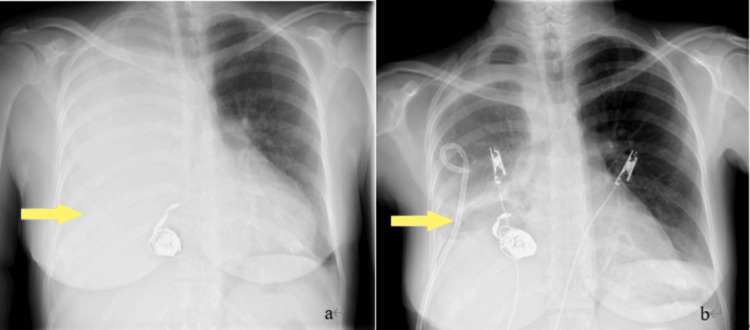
Postoperative chest radiographs (a) Ten days after surgery, mediastinal shift is observed. (b) Three days after inserting a pigtail drainage catheter, improvement is observed.
